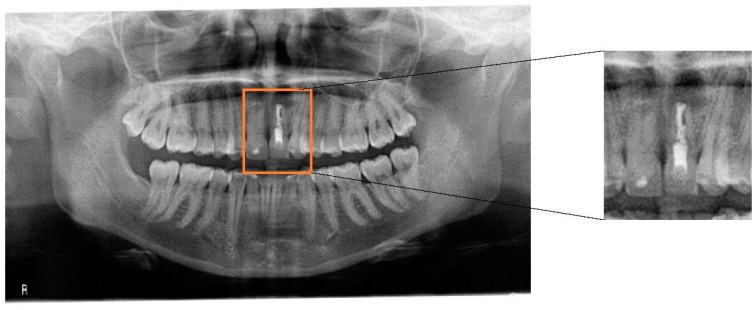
Panoramic radiograph of the patient. A radiolucid periapical lesion on tooth 21 can be observed, which did not disappear after endodontic treatment and apicoectomy.
